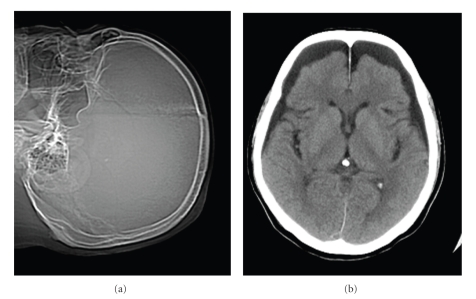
One month postoperative head image ((a): Plain radiograph, (b): CT). (a) Plain radiograph demonstrates the disappearance of intracranial air. (b) CT one month after surgery demonstrates the disappearance of air in the overall space. (c) CT: Computed tomography.
